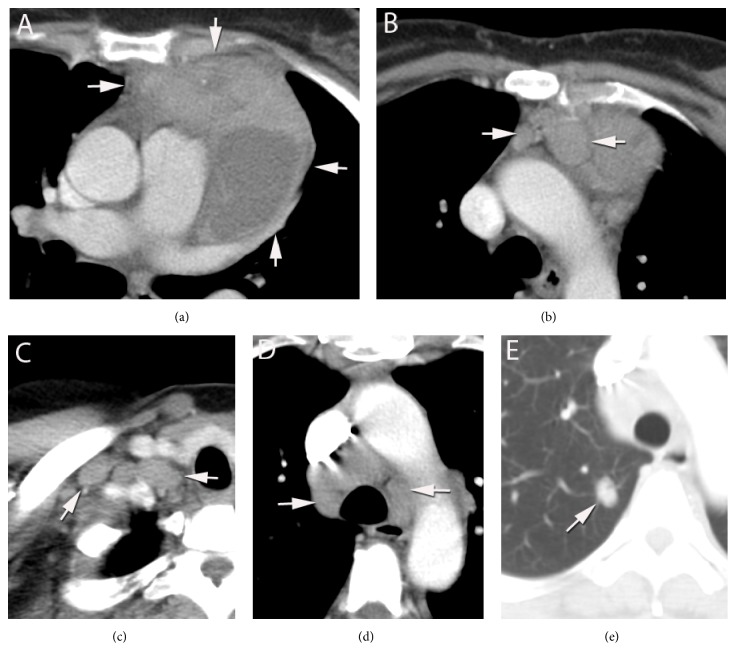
Chest computed tomography (CT) scan. Before resection of the mediastinal mass, chest CT scan showed a 12 cm anterior mediastinal mass (a) and lymph nodes (b). A year after the surgery, CT scan revealed multiple enlarged lymph nodes, such as cervical lymph nodes (c), paratracheal lymph node (d), and pulmonary nodules (e). Arrows indicated the lesions.
